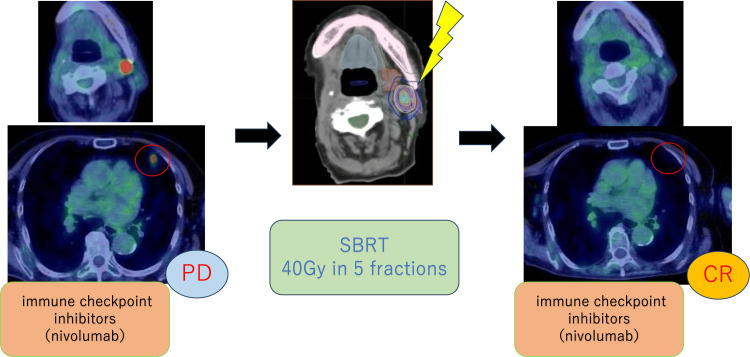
First abscopal effect observed after SBRT to cervical lymph node metastasis PET-CT images of the first abscopal effect. Three months after SBRT was performed for the left cervical lymph node metastasis, the metastatic lesion in the left lung S5 disappeared on PET-CT SBRT: stereotactic body radiotherapy; PET-CT: positron emission tomography-computed tomography
